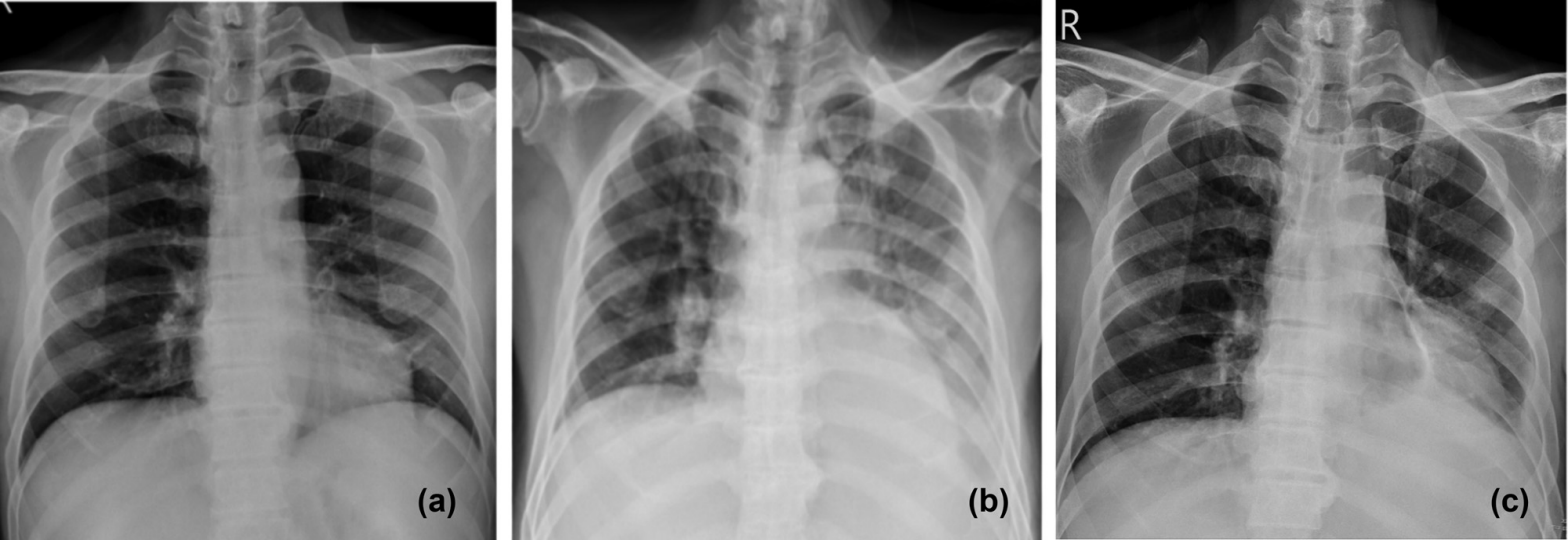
(a) Initial chest radiograph revealed no abnormal findings. (b) Chest radiograph taken revealed rapid accumulation of a left side pleural effusion within 1 week of admission. (c) Pleural effusion largely resolved after induction chemotherapy.
